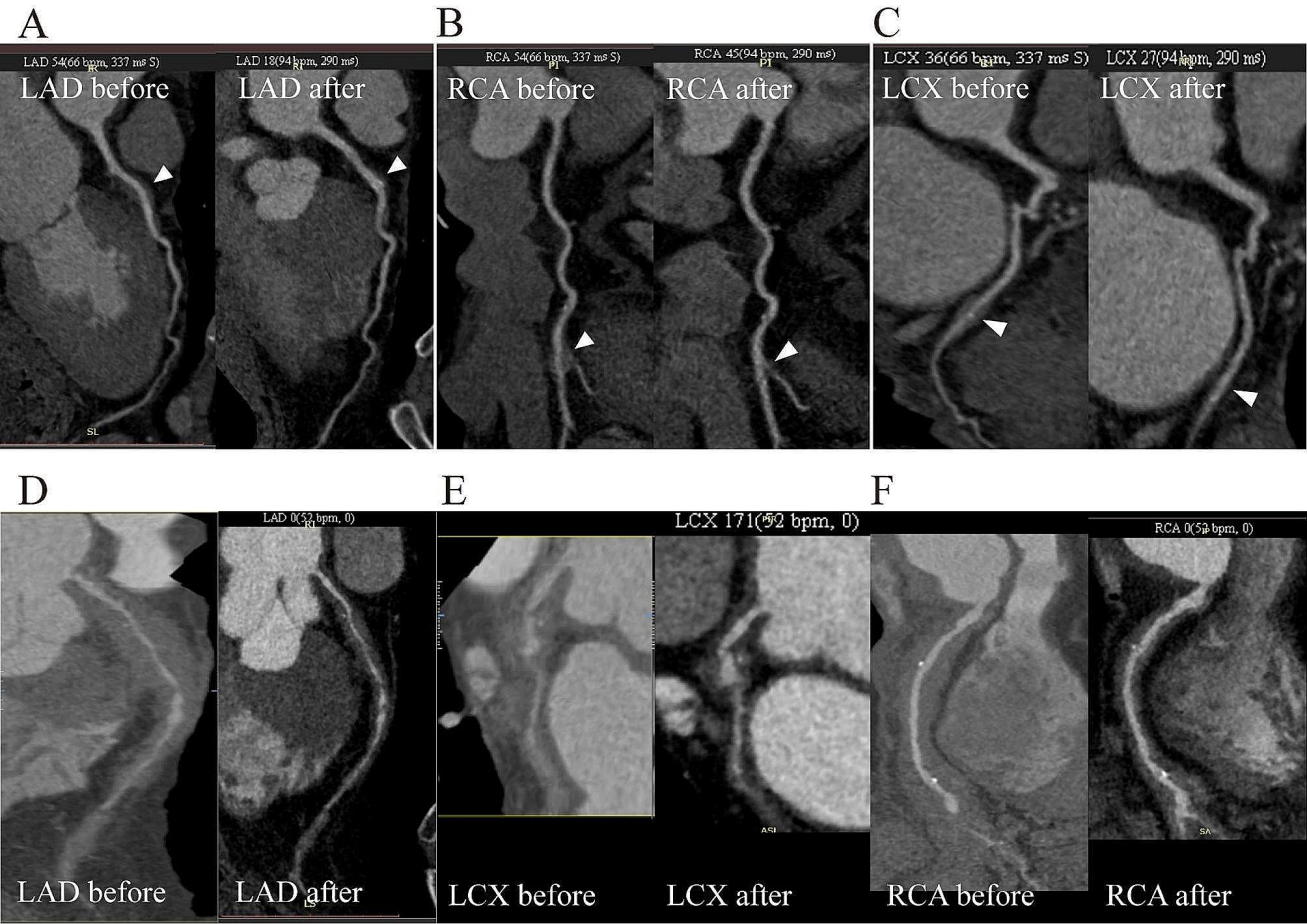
(A-C) Curved multiplanar reformatted coronary artery CTA demonstrate focal mural thickening and lumen stenosis (triangle) of left anterior descending artery (LAD), right coronary artery (RCA) and left circumflex artery (LCx) in a 59-year-old man before and after treatment. (D-E) Curved multiplanar reformatted coronary artery CTA demonstrate diffuse mural thickening and lumen stenosis of LAD, LCx and RCA in a 69-year-old man before and after treatment
